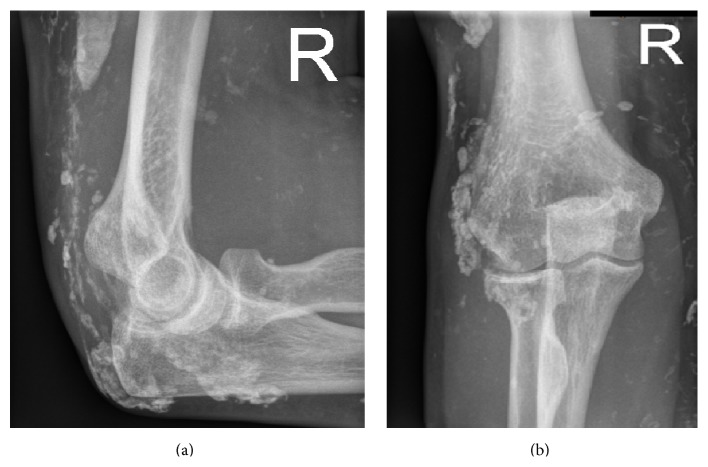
Standard elbow radiographs of a 24-year-old woman six weeks after cautious broaching of large calcific lesions in calcinosis universalis associated with juvenile Dermatomyositis of the musculus triceps brachii and its tendon plus resection of an ulcerating sinus on (a) anterior-posterior radiograph, (b) lateral radiograph.
